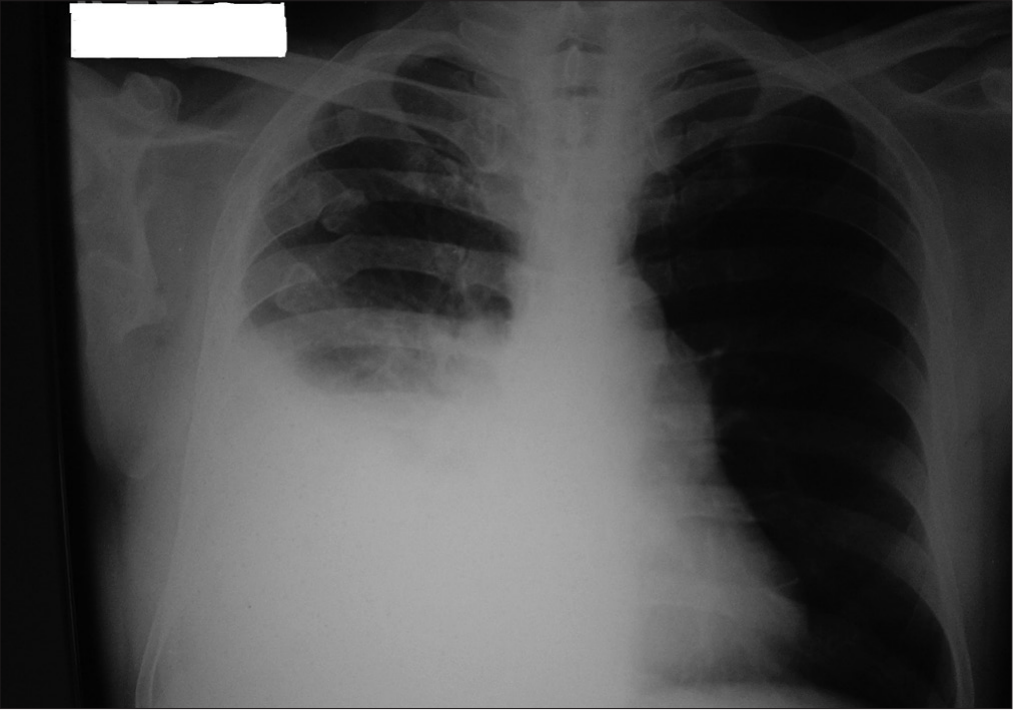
Chest X-ray on admission shows right pleural based
